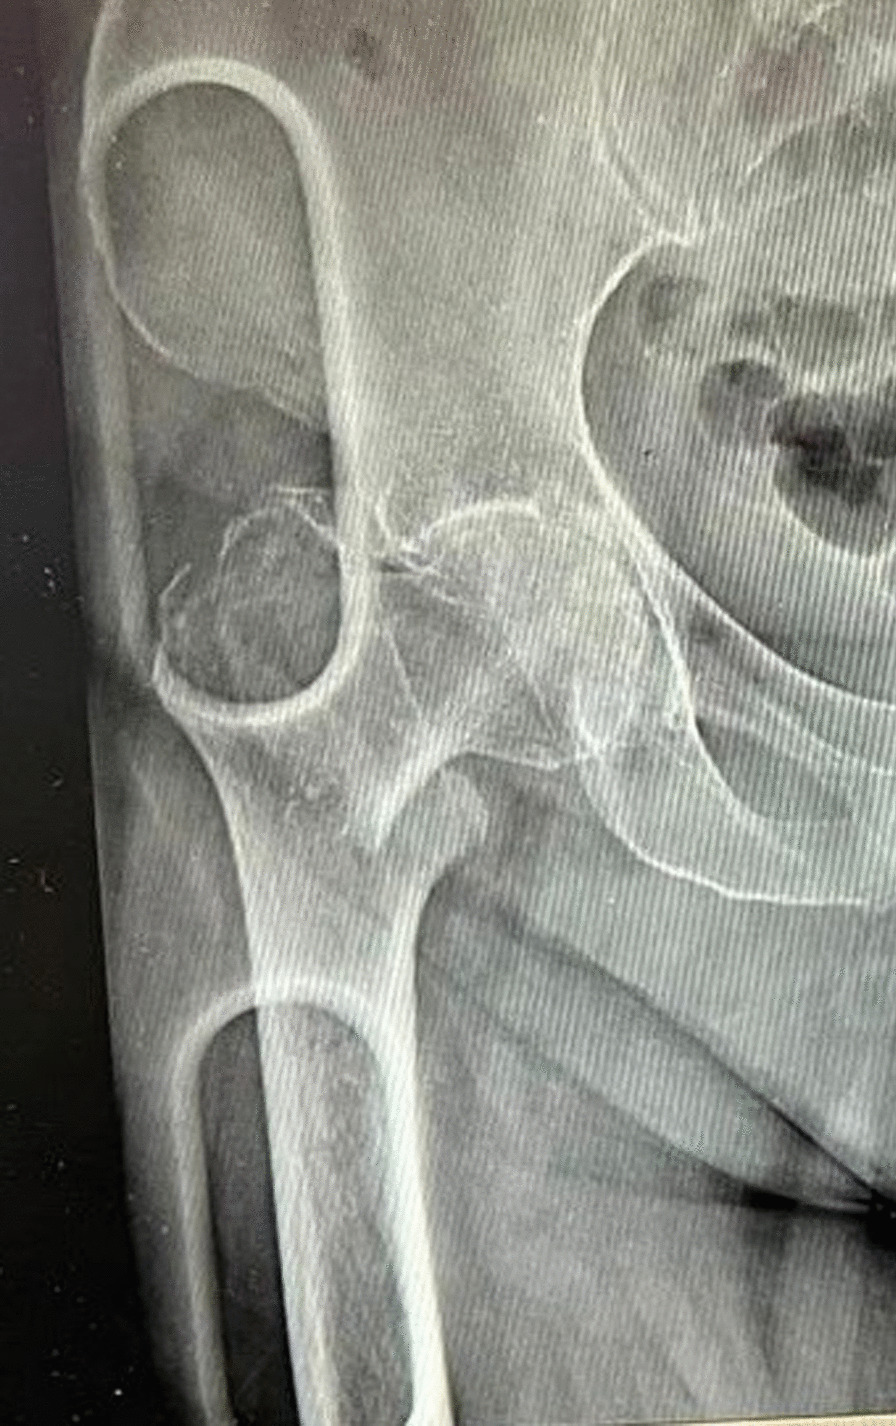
Preoperative anteroposterior radiograph of the right hip demonstrating displaced intertrochanteric femur fracture
